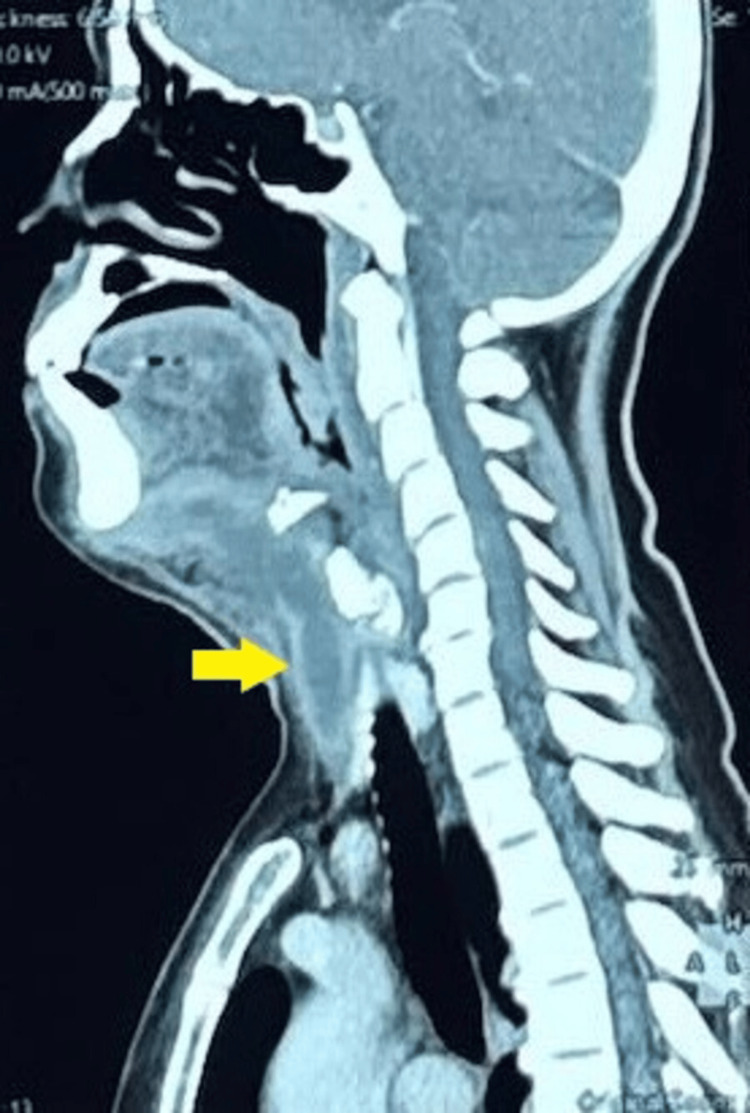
CT of the neck showing multiloculated communicating right cervical collections in the right parapharyngeal and right submandibular spaces, indicating abscess formation (indicated by arrow)
